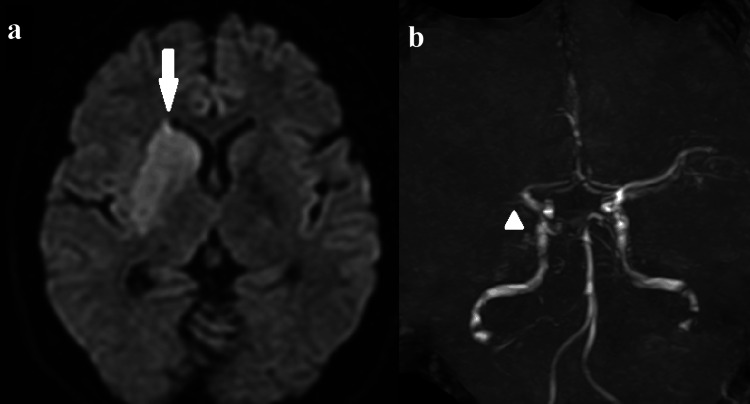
Initial MRI scan shows (a) high intensity area on the right basal ganglia and (b) the occlusion of right proximal middle cerebral artery.
